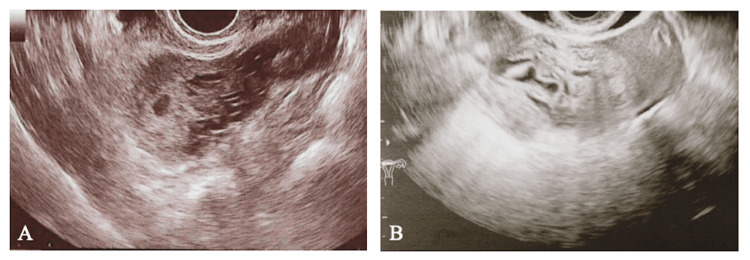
Transvaginal ultrasonography (A) Findings from the previous clinic: a hypoechoic 4 cm mass thought to be a gestational sac was observed in the right uterine horn; (B) Findings from our hospital: a heterogeneous 4 cm mass was observed in the right uterine horn.
